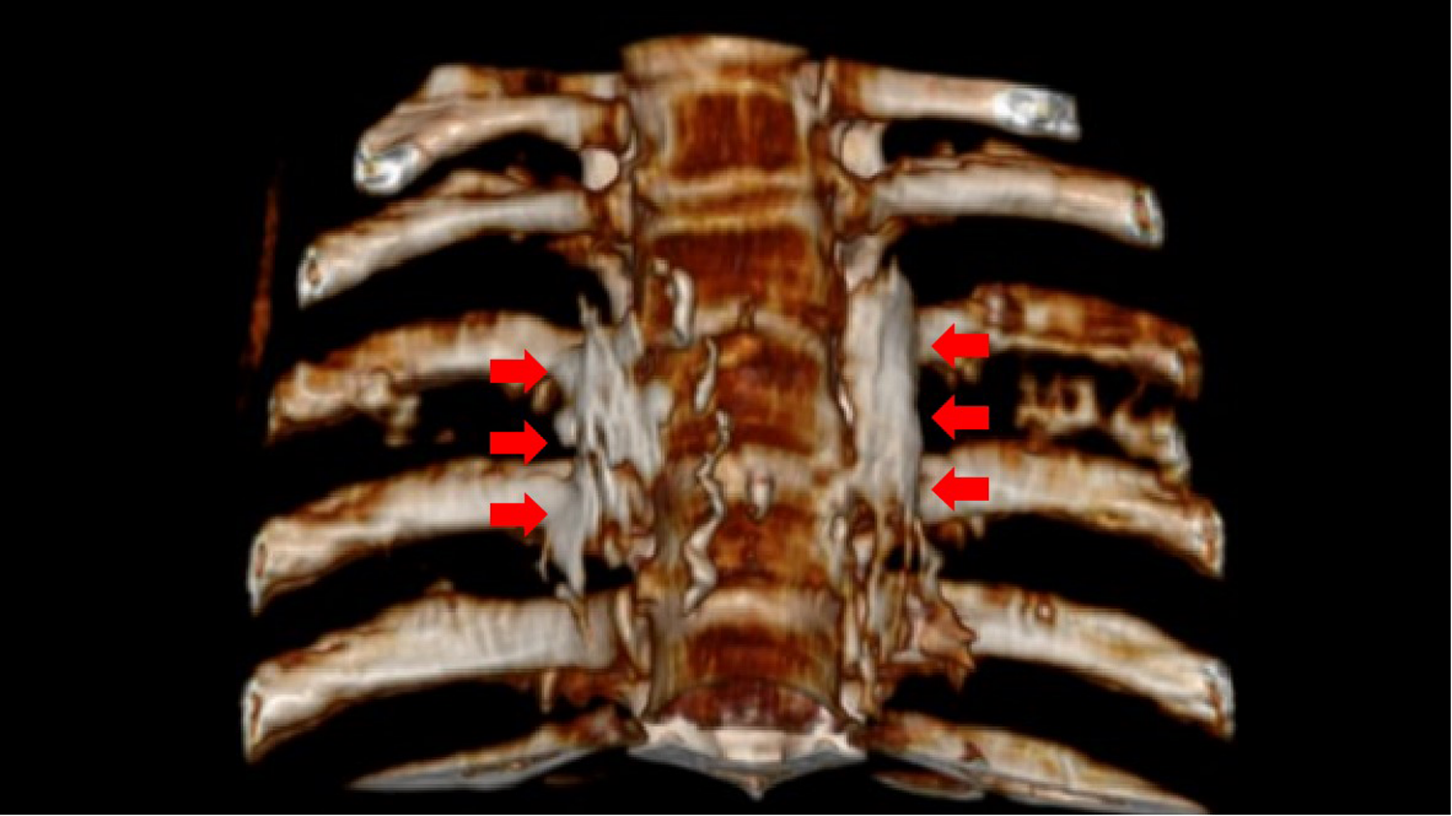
The distribution of absolute alcohol on both sides after three-dimensional CT reconstruction, which wraps around the small head of the 4th rib and the lateral edge of the 4th vertebral body, as indicated by the red arrow in the figure.
